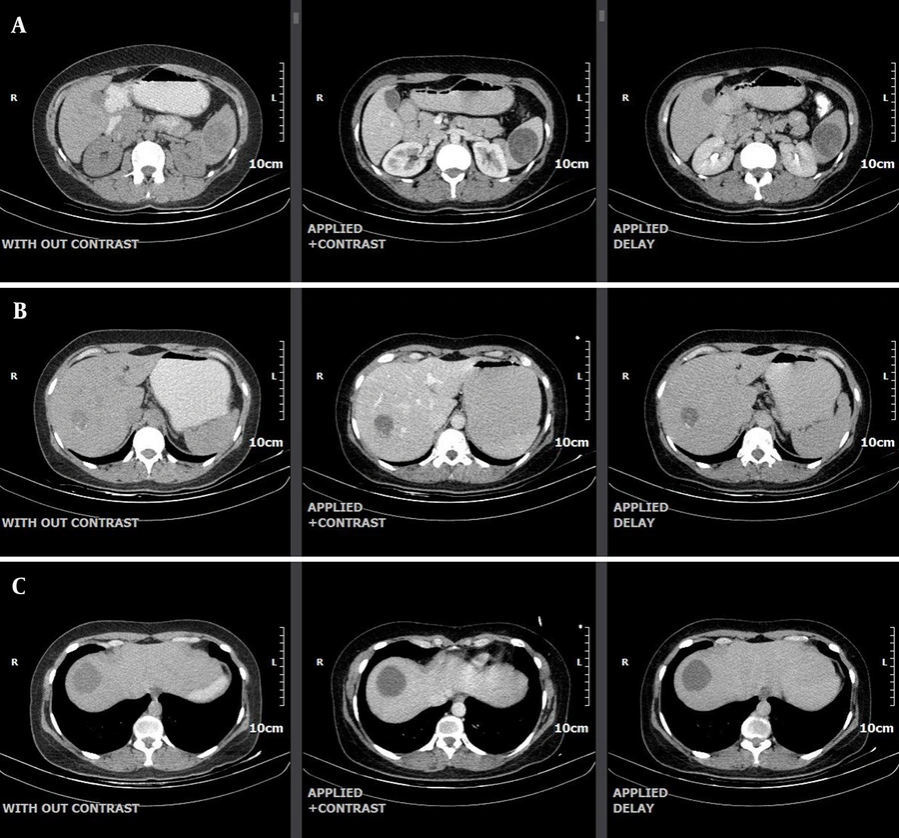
A, computed tomography (CT) images demonstrate a hydatid cyst measuring 43 mm in diameter in the spleen; B, computed tomography (CT) images demonstrate a hydatid cyst measuring 32 mm in diameter in segment 7 of the liver; C, computed tomography (CT) images demonstrate a hydatid cyst measuring 38 mm in diameter in segment 8 of the liver.
